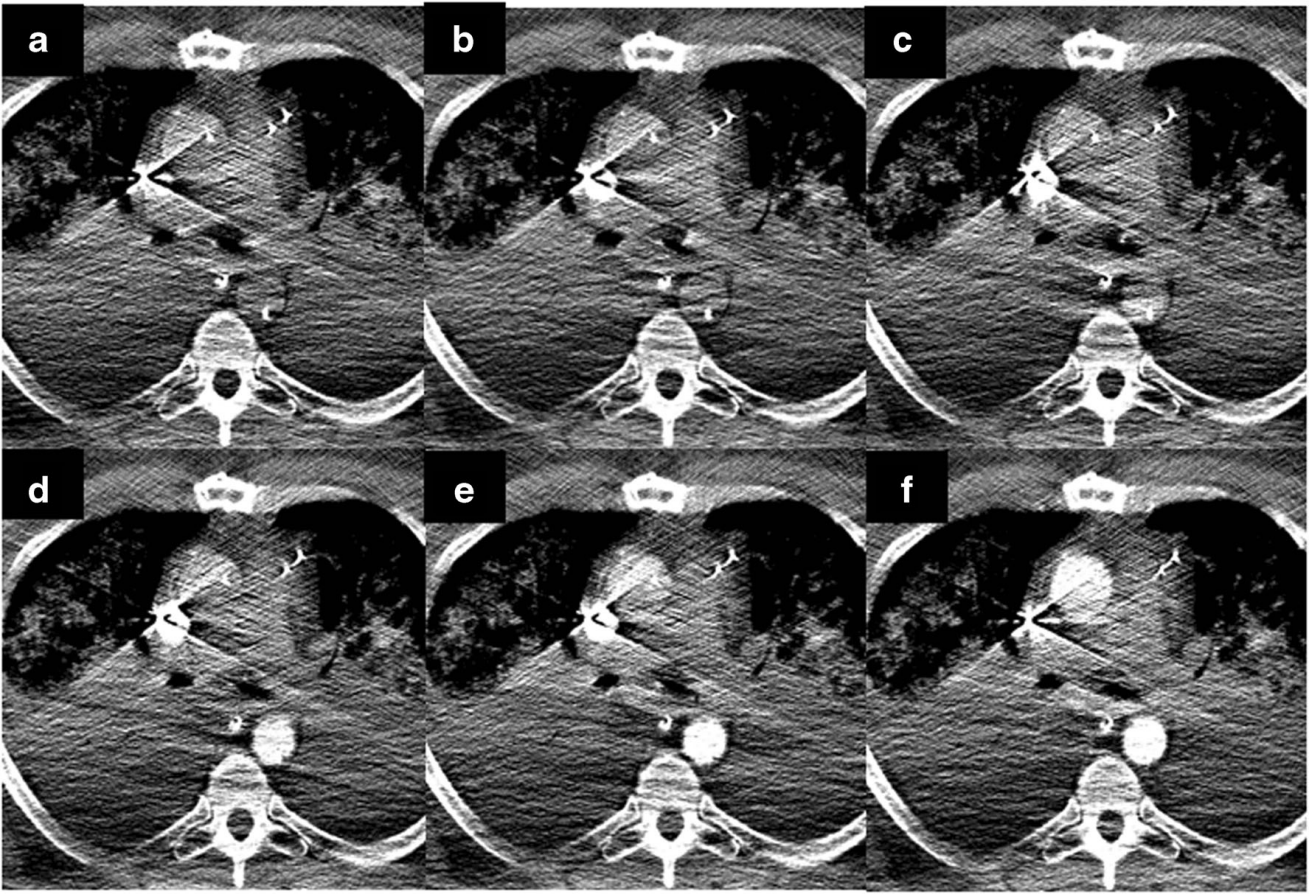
Sequential axial CT images (a–f) from a test bolus obtained at the level of main pulmonary artery. Notice the altered pattern of contrast flow. In a normal patient, contrast injected from the upper extremity first opacifies the SVC, followed by the right atrium, right ventricle, pulmonary artery, pulmonary vein, left atrium, left ventricle, ascending aorta, and finally the descending thoracic aorta. In this case we see an altered pattern of contrast flow: first SVC, followed by minimal opacification of the pulmonary artery and ascending aorta, dense opacification of the descending aorta followed by dense opacification of the ascending aorta. There is insufficient opacification of the pulmonary circulation due to siphoning of contrast by ECMO
